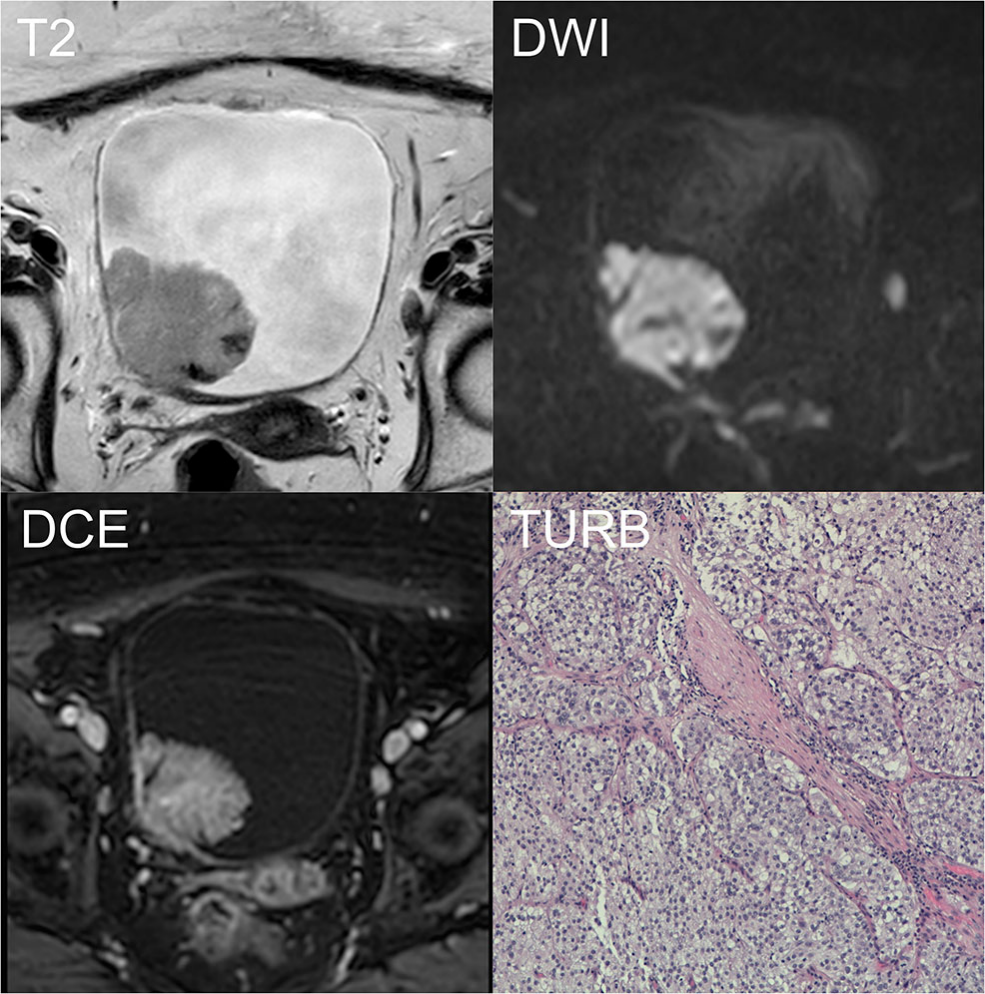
Example of correctly classified muscle-invasive BC. A 90-year-old woman with hematuria and a bladder mass reported after flexible cystoscopy underwent mp-MRI before primary TURB. T2W imaging (T2) showing an exophytic lesion on the right lateral wall, > 1 cm in the major axis with focal interruption of the SI of the muscularis propria. The *b*1000-DWI (DWI) confirming the high signal intensity of the tumor extending to the muscular layer. Based on bp-MRI, all readers assigned a VI-RADS category of 4. DCE imaging (DCE) showing early enhancement of the lesion and inner layer with an early enhancement of the muscularis propria, indicating tumor infiltration. DCE did not affect the VI-RADS category. T stage after TURB was HG-T2 (TURB). DCE, dynamic contrast-enhanced; DWI, diffusion-weighted imaging; HG, high grade; mp-MRI, multiparametric magnetic resonance imaging; bp-MRI, biparametric magnetic resonance imaging; SI, signal intensity; T2W, T2-weighted; TURB, trans-urethral resection of the bladder; VI-RADS, Vesical Imaging-Reporting and Data System
